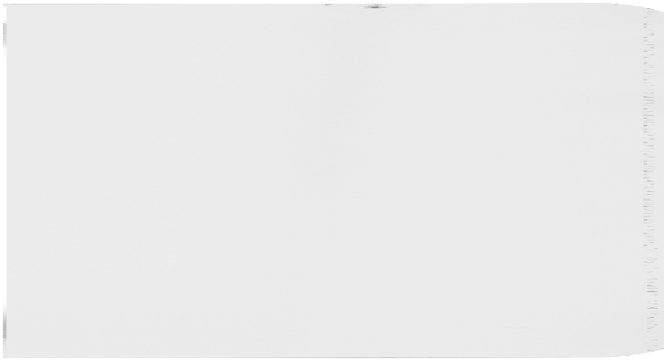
Radiography film image when the film is exposed to the 12.0 wt% of nominal uranium-235 fuel when the shutter is closed.
